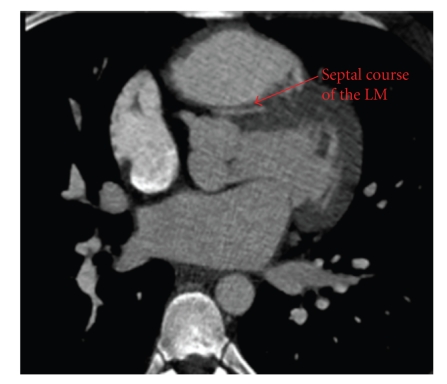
Coronary CT showing the left main (LM) traveling between the left ventricular outflow tract (LVOT) and the right ventricular outflow tract (RVOT).
